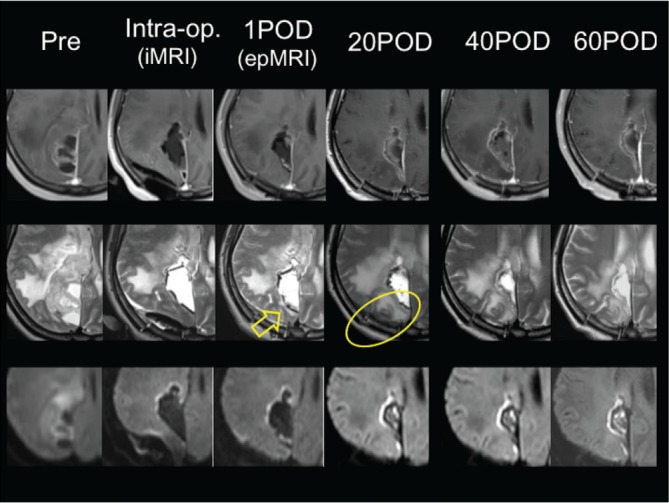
Gadolinium (Gd)-enhanced T_1_-weighted imaging (T_1_WI) (upper images), T_2_WI (center row of images) and diffusion-weighted imaging (DWI) (lower images) of a representative carmustine (BCNU) wafer-implanted case (43 y.o. woman, secondary glioblastoma with an oligodendroglia component) with slight early postoperative high-intensity area on T_2_WI (ep-T_2_-HIA) expansion (a depth of 6.1 mm) in the adjacent parenchymal area without markedly restricted diffusivity (yellow arrow). The T_2_-HIA on early postoperative magnetic resonance imaging (ep-MRI) changes to delayed brain edema 20 days after surgery (yellow circle).
